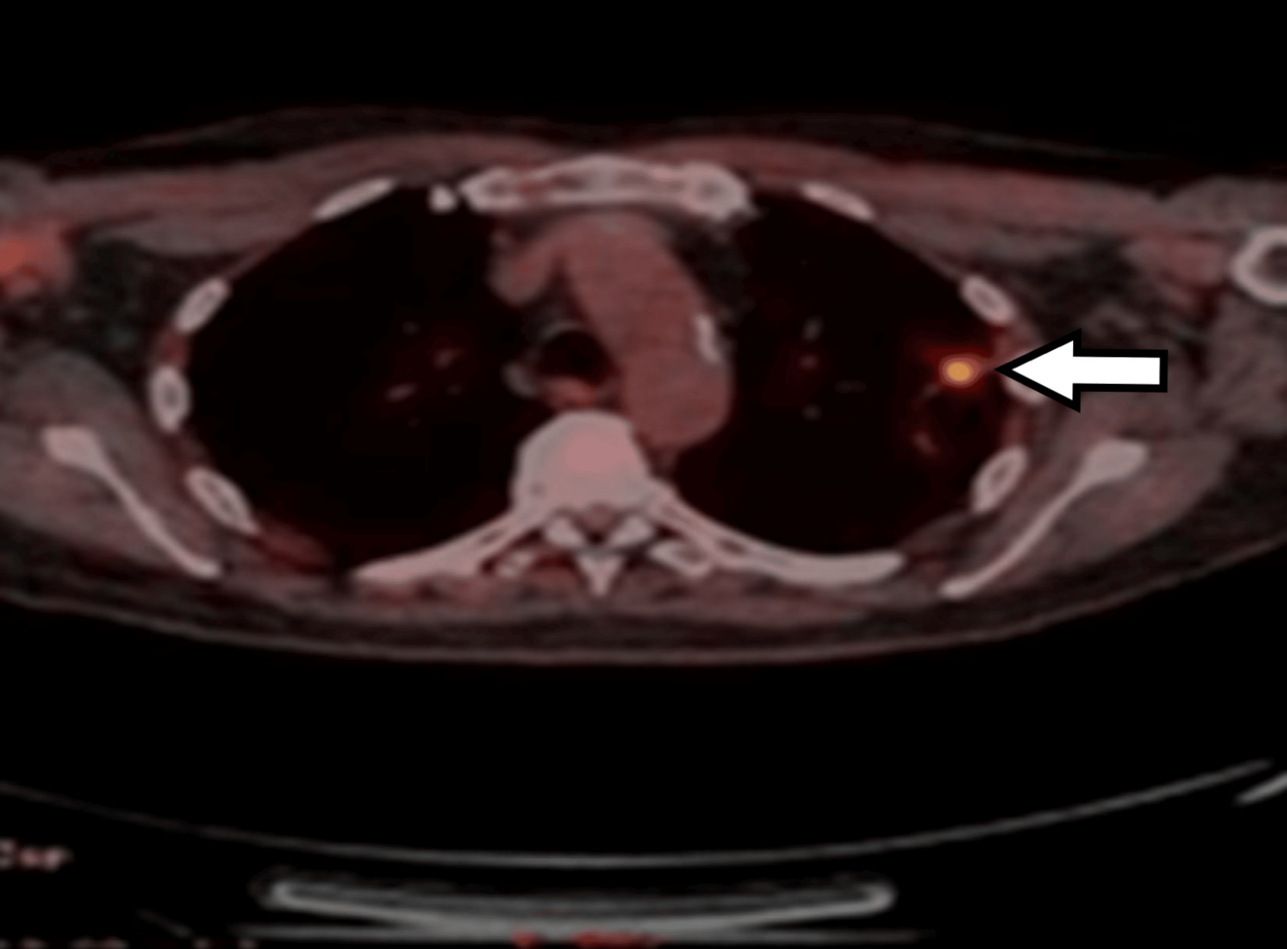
Axial PET scan of the chest demonstrating moderate FDG uptake in a 17 mm soft tissue mass with associated atelectasis in the left upper lobe (white arrow) PET: positron-emission tomography; FDG: fluorodeoxyglucose
